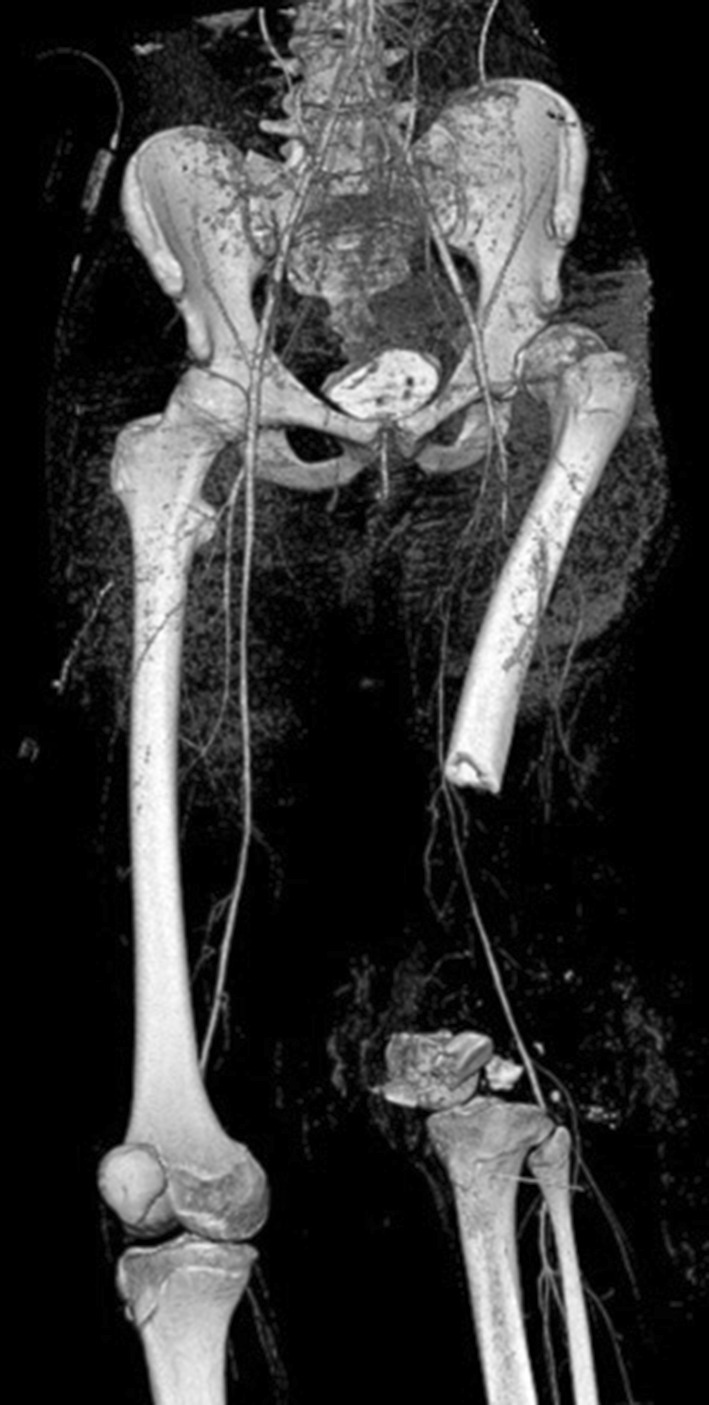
Computed tomography (CT) angiography demonstrates a complete dislocation of the left hip, segmental bone defect of approximately 26 cm of the distal femoral bone without signs of injury to the popliteal artery
